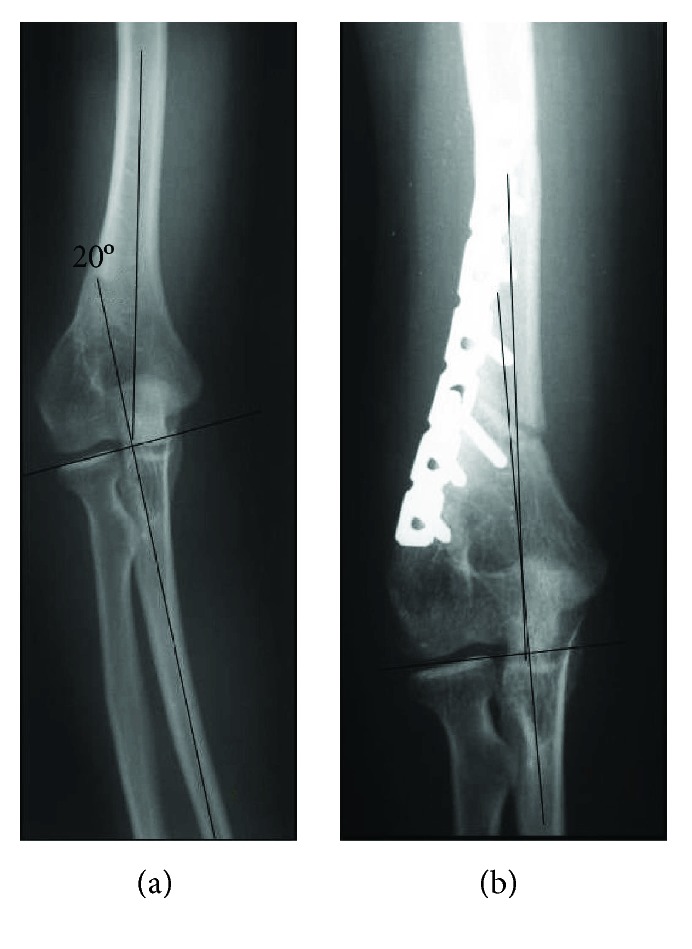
Case 1. (a) Preoperative AP radiograph. (b) Postoperative AP radiograph.
